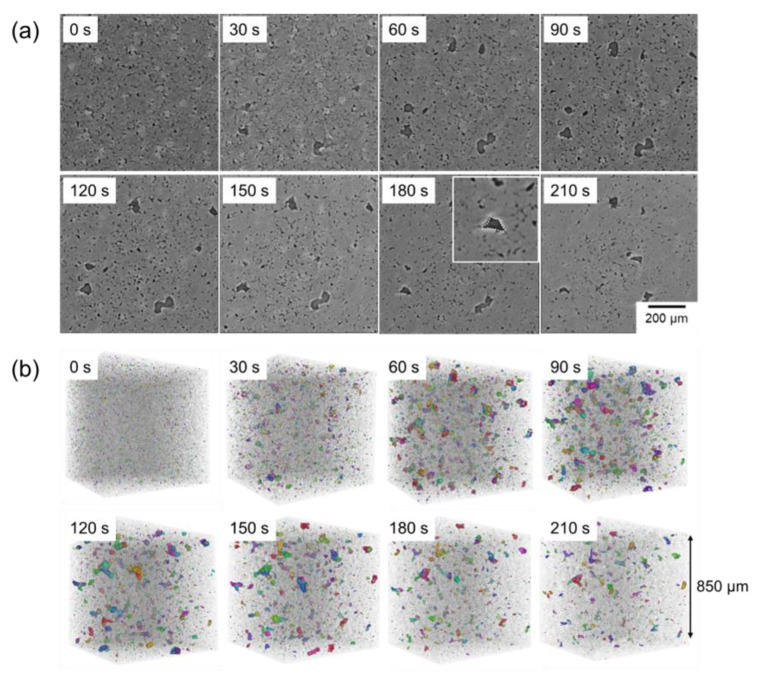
Synchrotron radiation X-ray CT images of (a) cross-sections and (b) three-dimensional pore distributions of the powder compact with an initial relative density of 90% obtained by continuous imaging at 660 °C for 0 s, 30 s, 60 s, 90 s, 120 s, 150 s, 180 s, and 210 s. In the cross-sectional image at 180 s, the curvature at the solid/pore interface is also shown.
